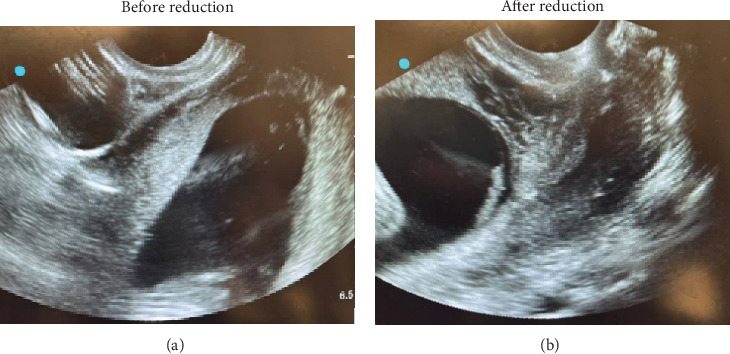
Bedside ultrasound imaging of the uterus. (a) Ultrasound reveals retroverted incarcerated gravid uterus. (b) After manual reduction resolving the incarceration (this study (Nguyen et al., 2025, “Management of Acute Urinary Retention due to Bladder Outlet Obstruction in the Setting of Incarcerated Gravid Uterus”. Case Reports in Urology)).
